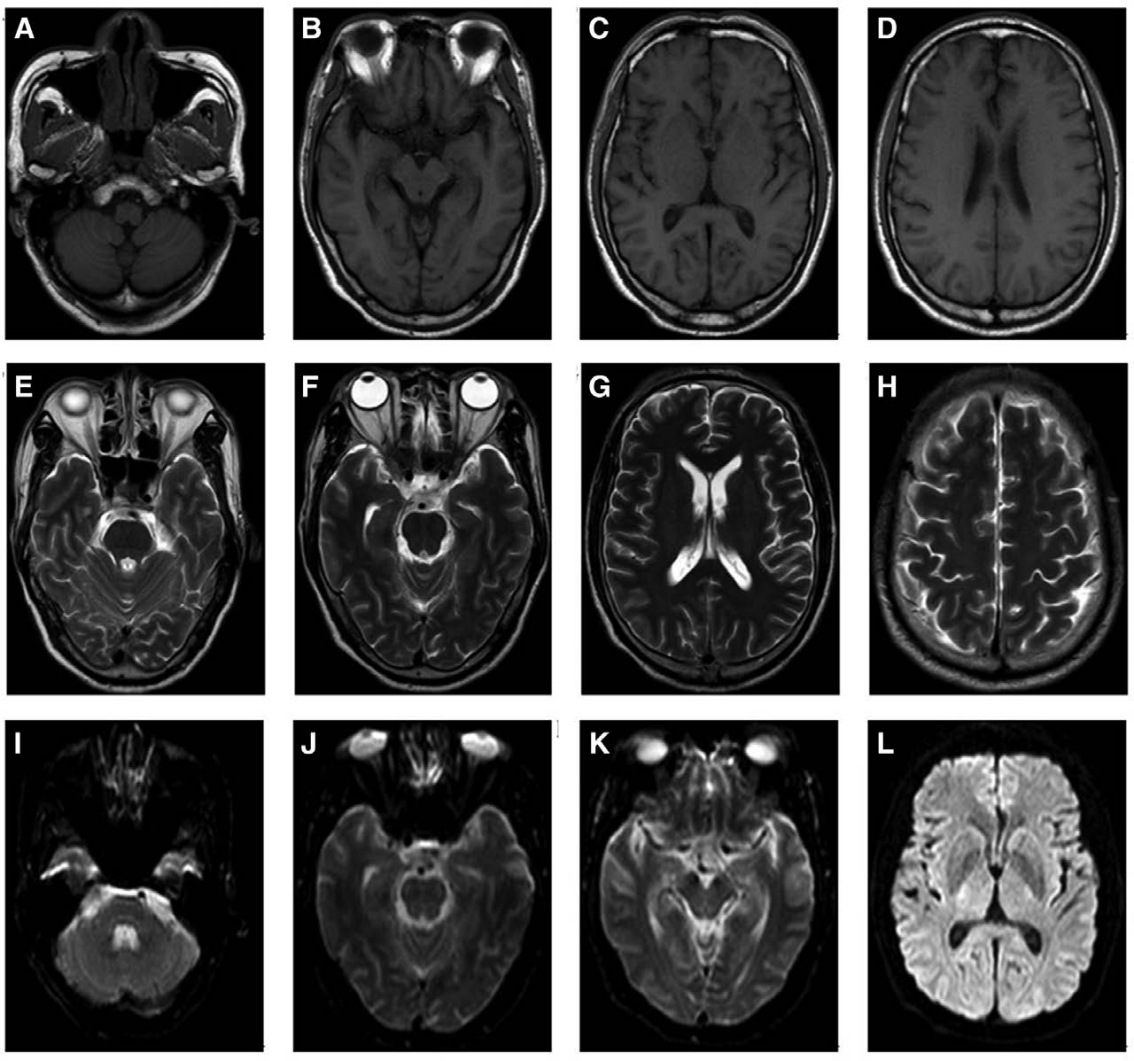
The changes in cranial MRI in the patient. MRI = nuclear magnetic resonance.
